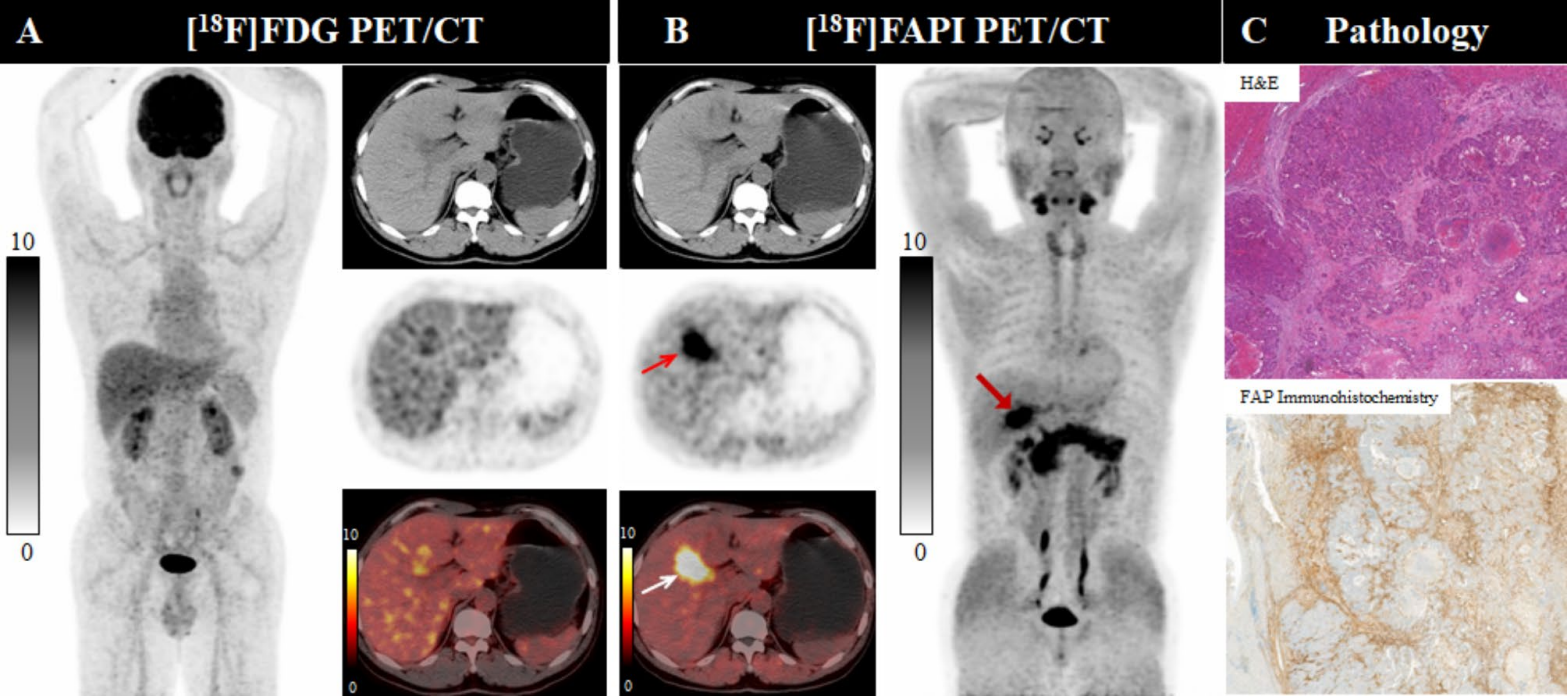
A 57-year-old male patient (Patient No. 4) with intrahepatic cholangiocarcinoma (ICC) was confirmed by postoperative pathology. [^18^F]FDG PET/CT (A) displayed no uptake in this lesion, conversely, [^18^F]FAPI-04 PET/CT (B) revealed intense uptake (SUV_max_ 13.7; TBR 11.4) in the hepatic segment IV/V lesion, as shown on both MIP images (large arrow) and axial images (small arrow). Pathological findings (C) indicate a heightened expression for FAP
